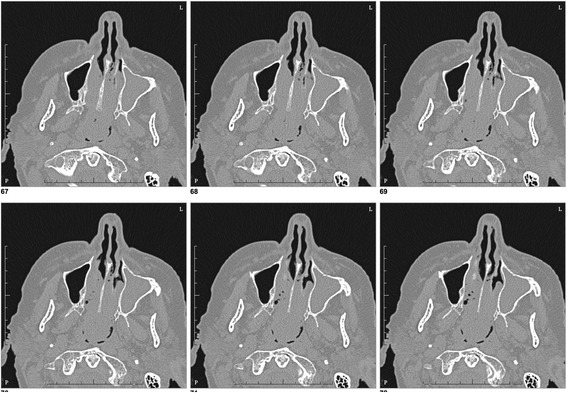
Computed tomography scan revealing a large soft tissue mass opacifying the left maxillary, ethmoidal, frontal, and sphenoid sinuses
